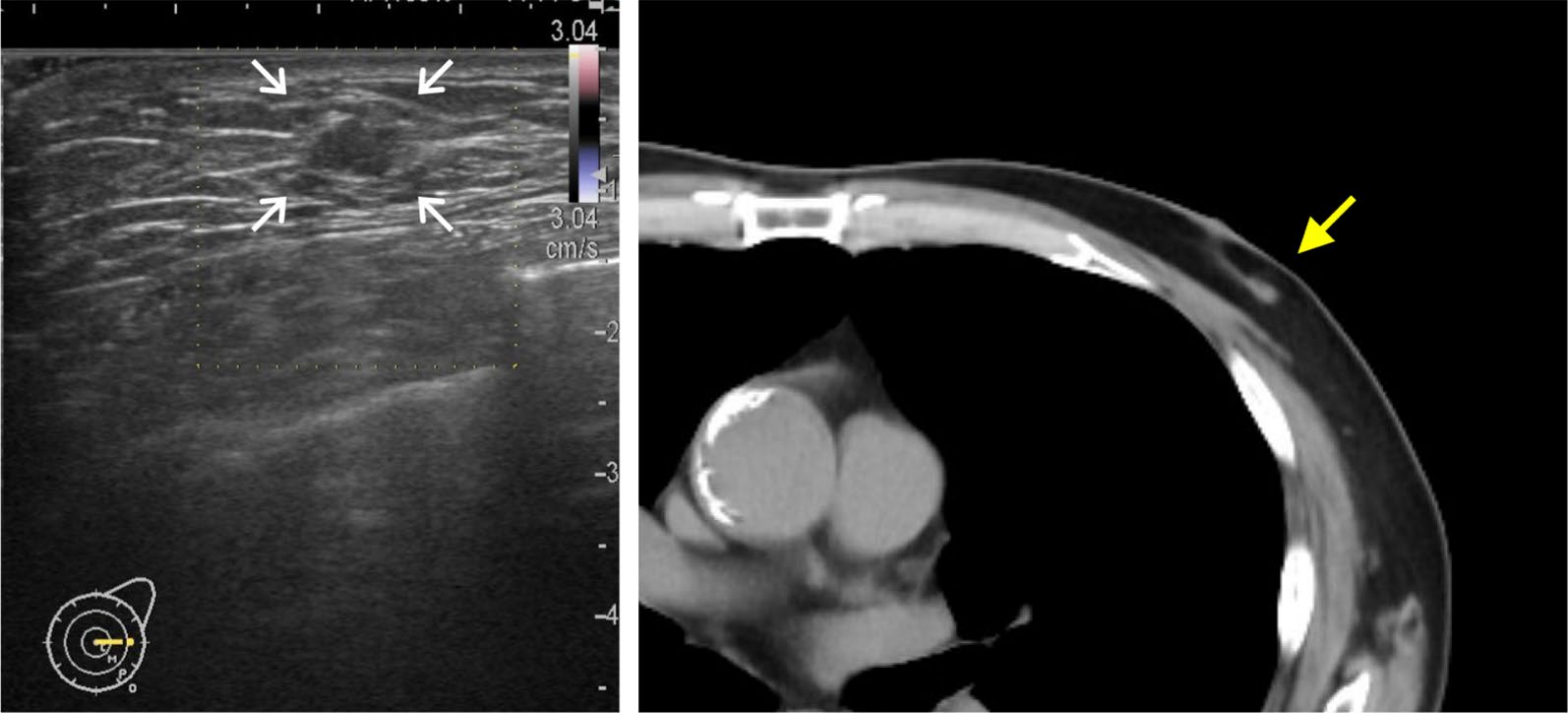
Imaging findings at 8 months after the disappearance of the tumor. Ultrasonography and CT revealed the reappearance of a 0.6-cm-diameter breast tumor in the same place as the previous mass
